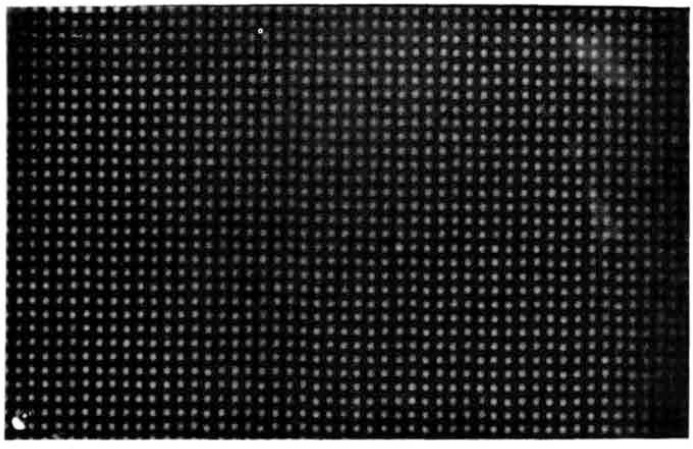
Radiograph of ^238^Pu large-area source number AA373 full scale.
